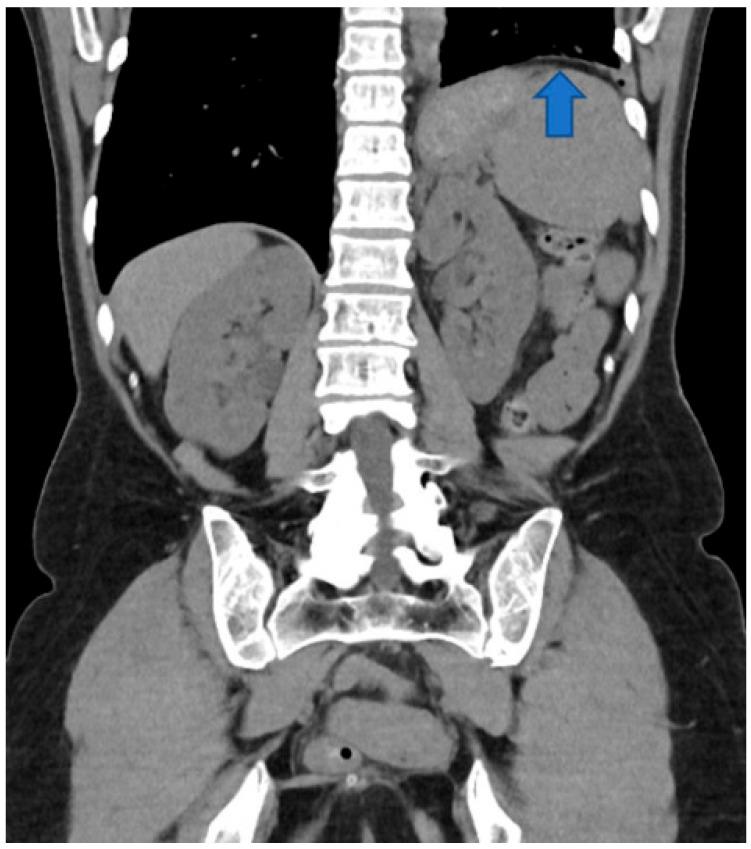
Coronal reconstruction of the unenhanced CT scan. The left diaphragm is abnormally elevated (blue arrow).
